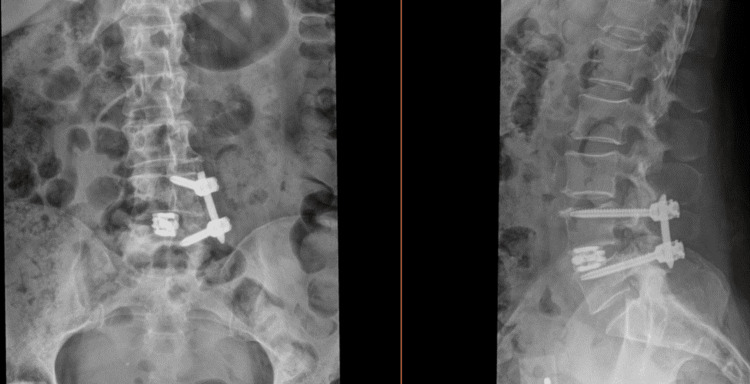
Postoperative (a) posterior and (b) lateral X-ray of left L4-5 pedicle screw fixation with interbody titanium graft
